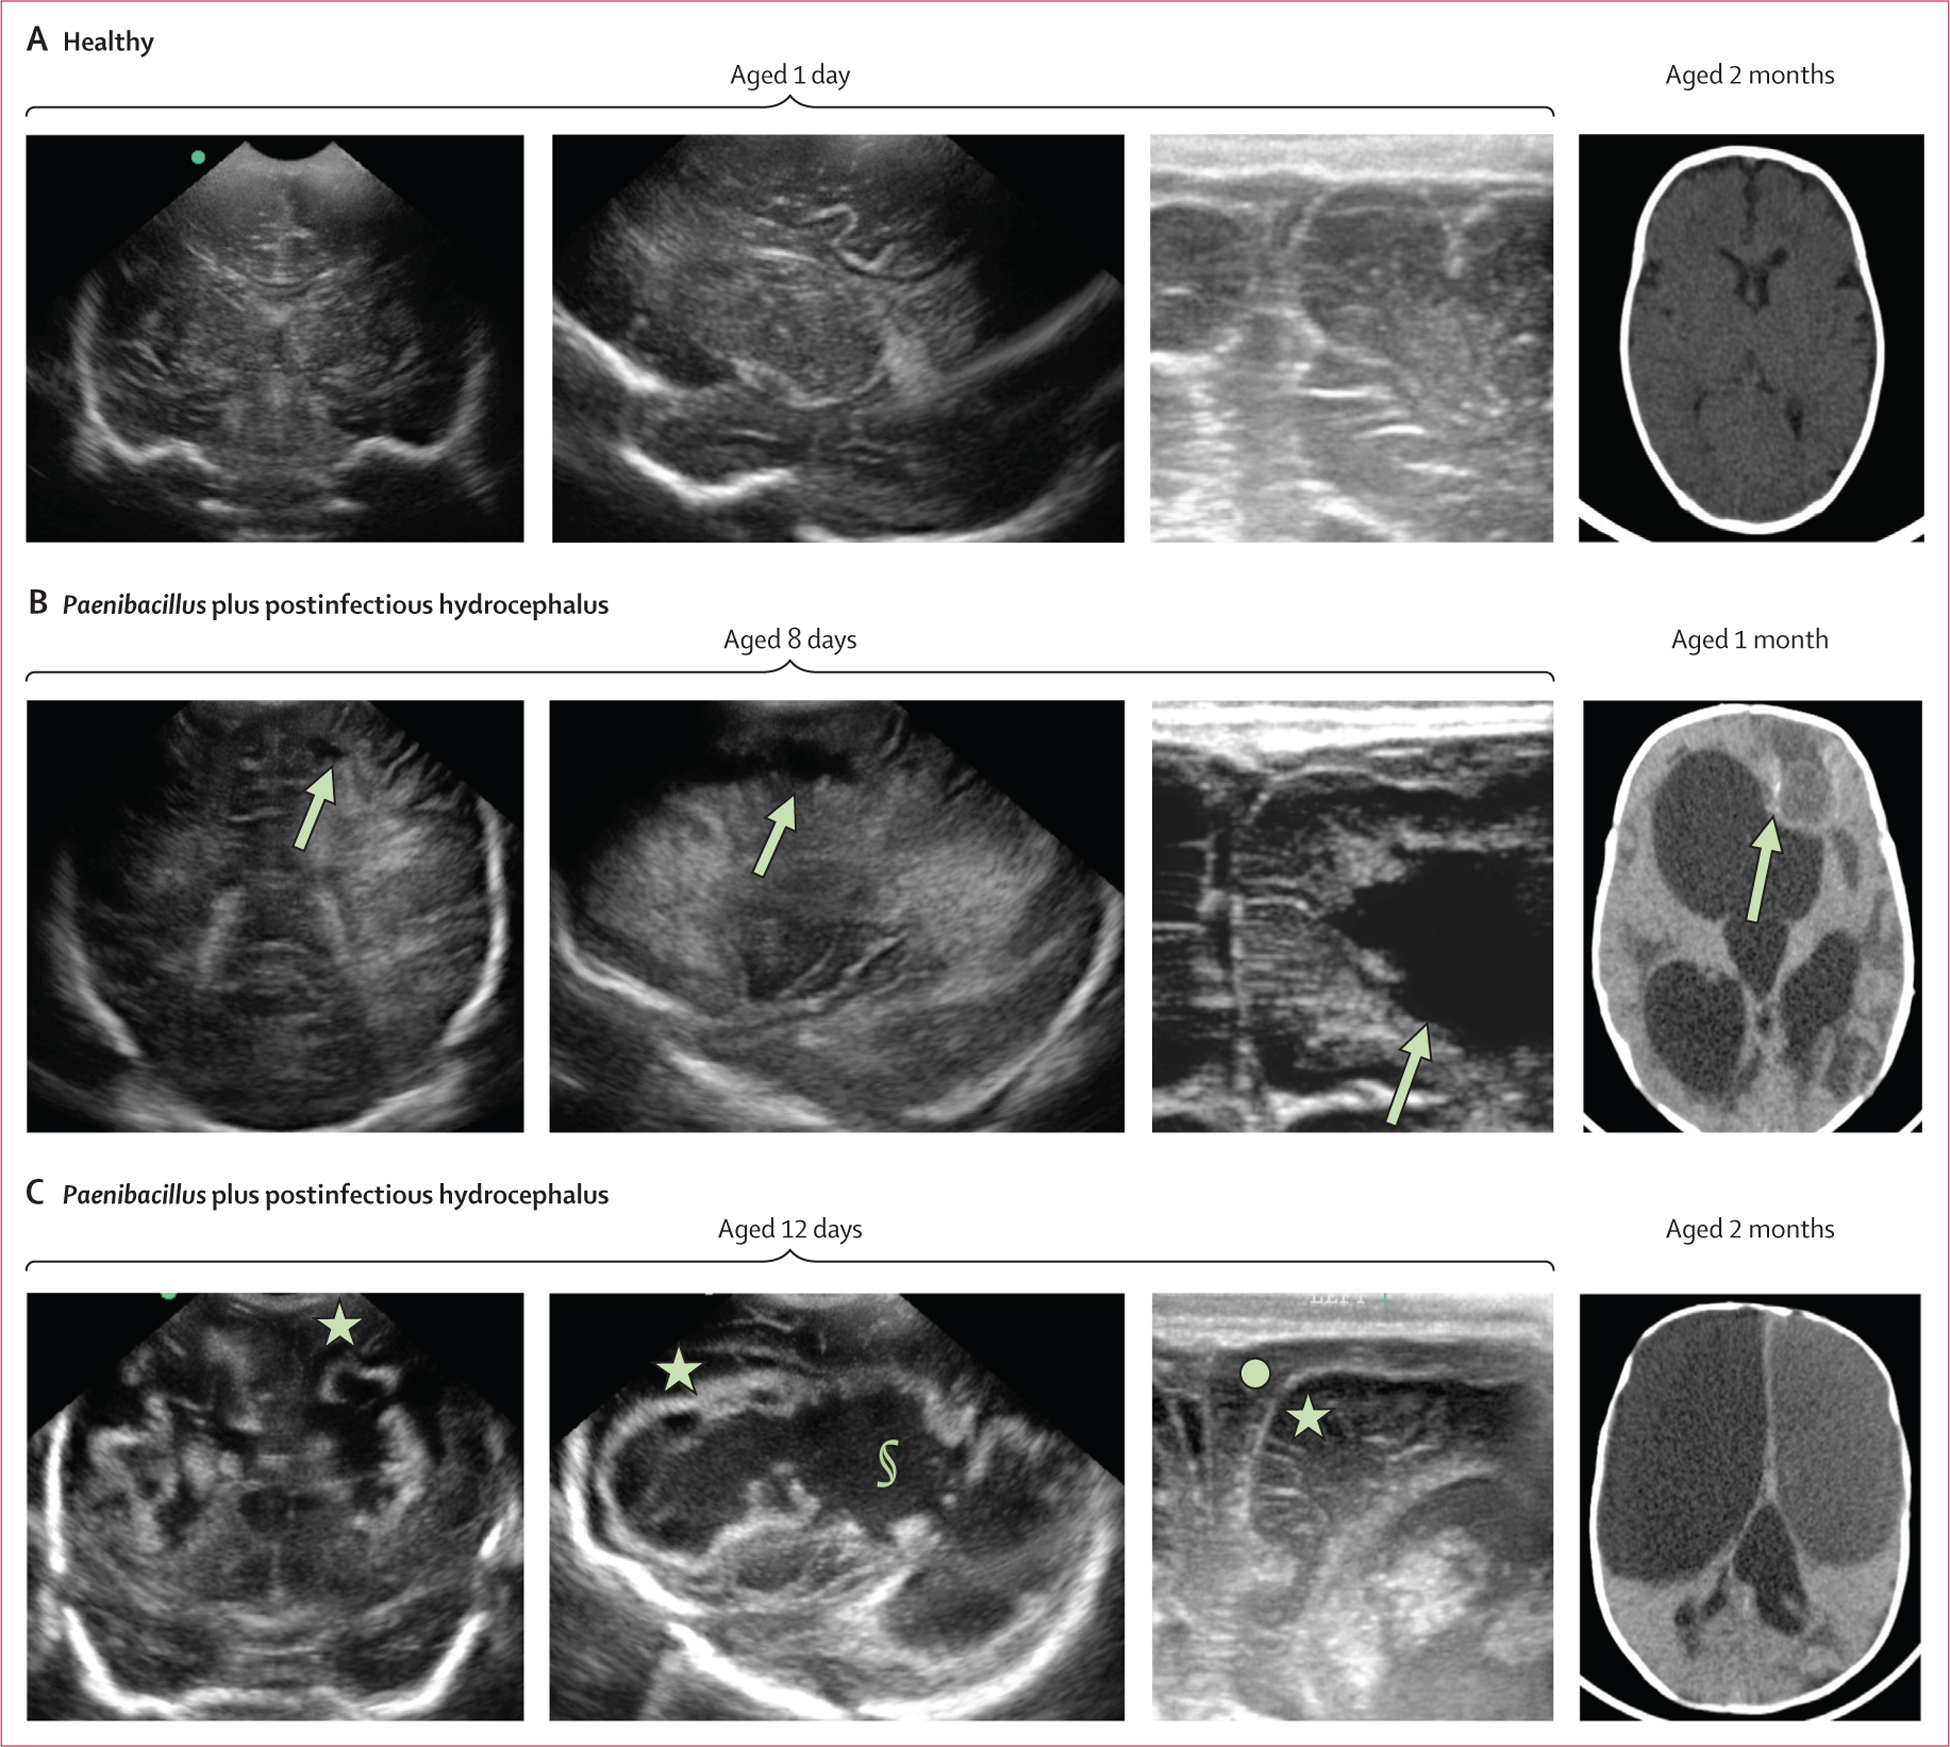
Imaging of neonatal *Paenibacillus thiaminolyticus* sepsis cases that subsequently developed postinfectious hydrocephalus 1 month=28 days. Representative cranial ultrasound from coronal, parasagittal and coronal views, and axial CT images, shown from left to right. A) Cranial ultrasound of a healthy neonate and CT image of a healthy 2-month-old infant are shown (reproduced from https://radiopaedia.org).^20^ B) 8-day old neonate (patient 1) with *P thiaminolyticus* CNS infection. Cranial ultrasound shows abnormal white matter and cortex echogenicity, extensive cortical and white matter injury from frontal to occipital lobes bilaterally, periventricular white matter injury, and a left frontal cortex and subcortical cystic lesion (green arrow). CT image of the same patient at 1 month before surgery for postinfectious hydrocephalus shows enlargement of the cyst with abscess formation (green arrow). C) 12-day-old neonate (patient 2) with *P thiaminolyticus* CNS infection. Cranial ultrasound shows cortical and subcortical white matter lesions with cystic evolution (white star), ventricular fluid with loculation, thickened hyperechogenic ventricular ependymal lining (S symbol), hyperechogenic gyri and sulci, and hyperechogenic extracerebral space (green circle). CT image of the same patient at 2 months of age before surgery for postinfectious hydrocephalus shows destruction of the bilateral frontal lobes, and residual ventricular debris.
